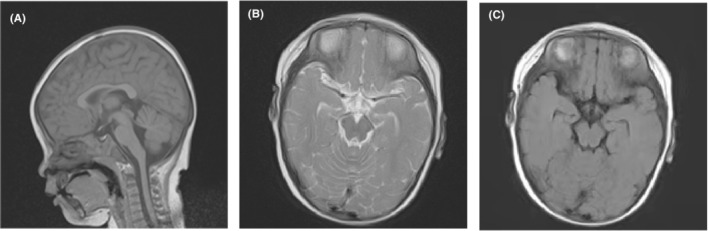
Magnetic resonance imaging of the brain of the proband showing normal findings without cerebellar or vermis atrophy. The sections are sagittal T1, axial T2, and axial fluid‐attenuated inversion recovery (from left to right).
